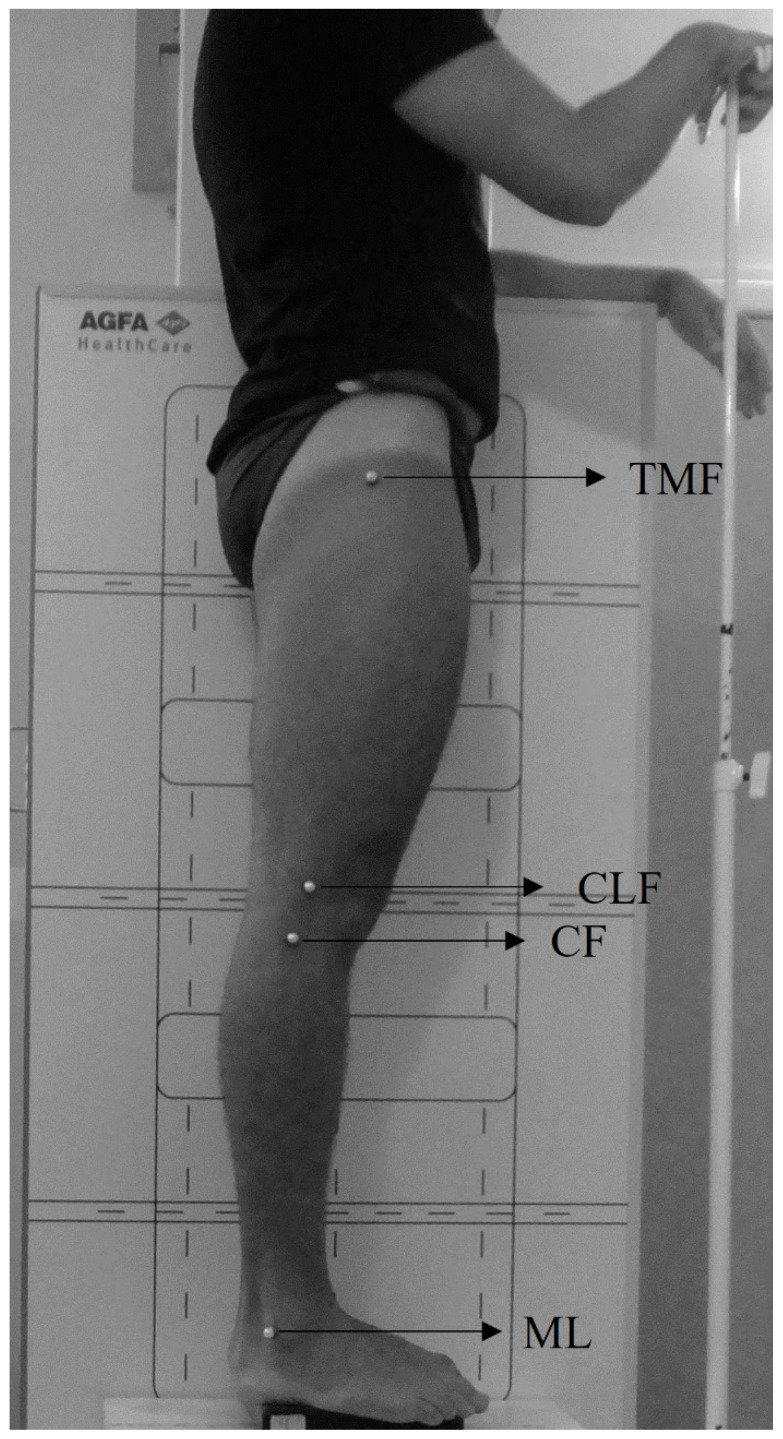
Anatomical landmarks used in the photogrammetric evaluation of the knee: GTF, LCF, HF, and LM. Positioning of participants for the photogrammetry and radiographic evaluations is also depicted.
